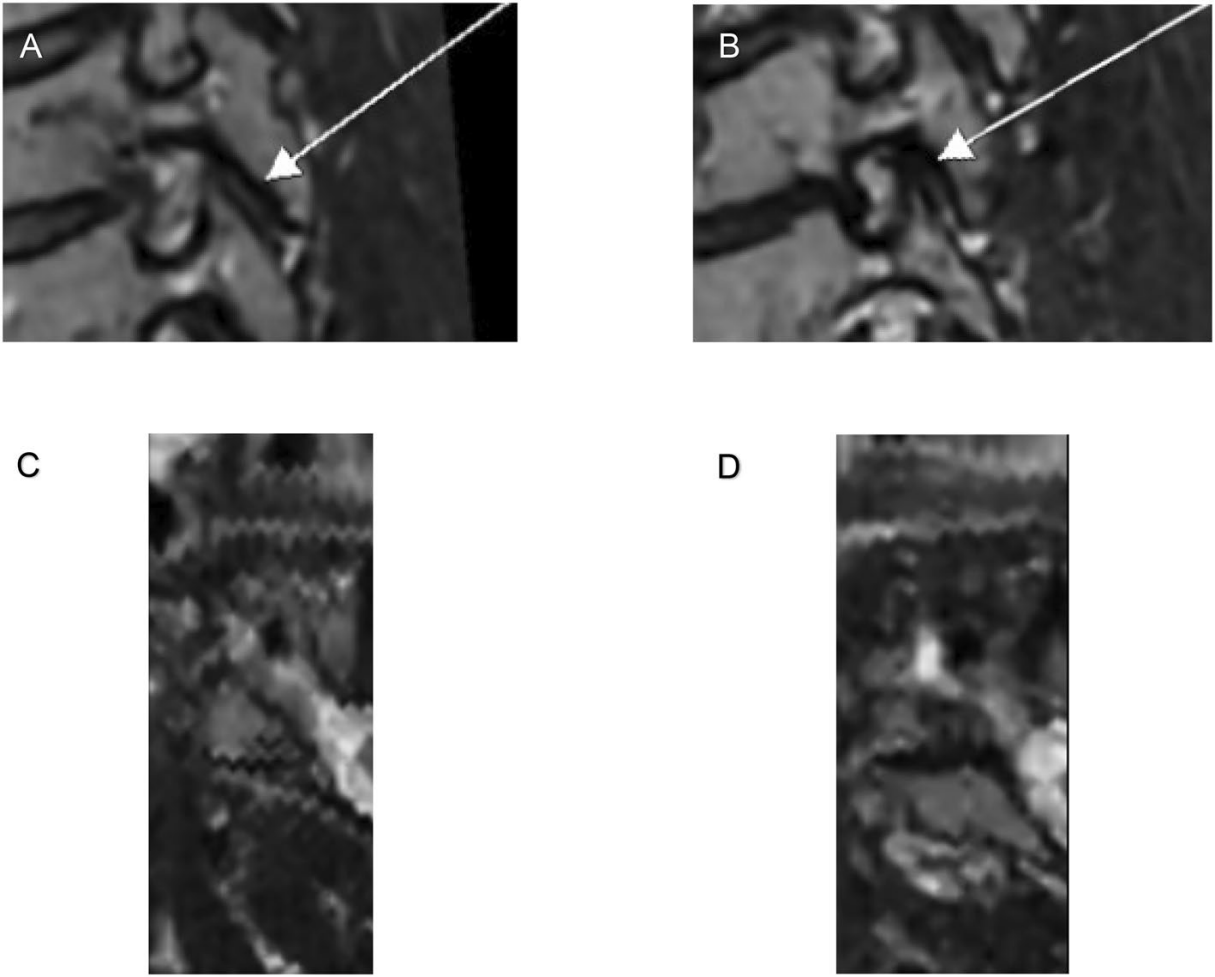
Magnetic resonance images of uncompressed and compressed foramina with the chosen sections demonstrating the smallest foraminal area/axial size for respective MR investigation. (A,B) depict the same subject and disc level before respectively after compression at level C4–C5 oblique sagittal plane, and in the axial plane (C,D).
